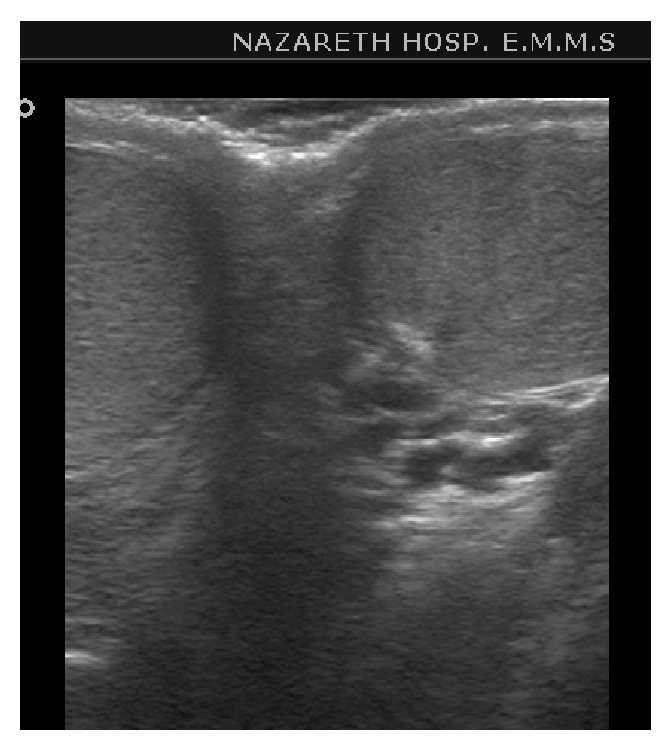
Transverse ultrasound of scrotum shows normal two testes in place.
